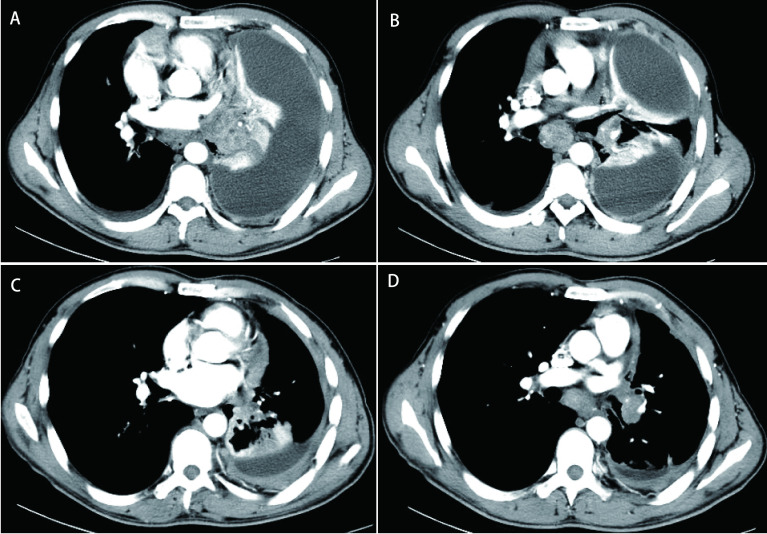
含培美曲塞方案化疗前及9个周期治疗后CT图像。A：化疗前CT图像(2015年8月17日)：左侧包裹性胸腔积液; B：化疗前CT图像(2015年8月17日)：纵隔淋巴结肿大，左肺下叶占位(3.8 cm×3.4 cm); C：9个周期化疗后CT图像(2016年3月24日)：左侧包裹性胸腔积液减少; D：9个周期化疗后CT图像(2016年3月24日)：纵膈肿大淋巴结缩小，左肺下叶占位缩小(2.6 cm×1.4 cm)。 CT images before and after 9 cycles of pemetrexed chemotherapy. A: CT images before chemotherapy (17-Aug-2015): left enveloped pleural effusion; B: CT images before chemotherapy (17-Aug-2015): mediastinal lymph node enlargement, left inferior lobe occupying space (3.8 cm×3.4 cm); C: CT images after 9 cycles of chemotherapy (24-Mar-2016): left enveloping pleural effusion was reduced; D: CT images after 9 cycles of chemotherapy (24-Mar-2016): mediastinal enlarged lymph node was reduced, left lower lobe area of the lung was reduced (2.6 cm×1.4 cm). CT: computed tomography.
